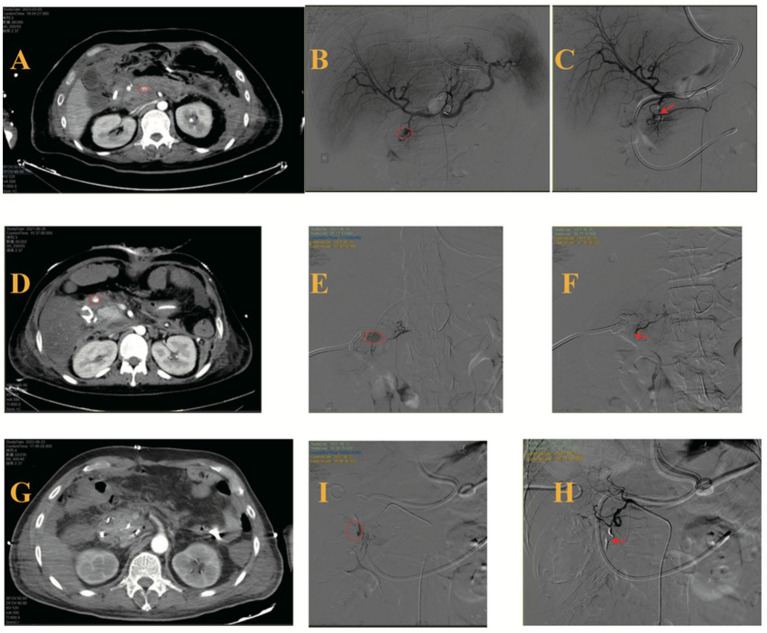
Representative imaging of severe acute pancreatitis (SAP)-associated hemorrhagic complications managed with digital subtraction angiography (DSA) and transcatheter arterial embolization. Red dashed circles indicate the bleeding sites, and red arrows indicate the embolization positions. Case 1: A 34-year-old man with idiopathic SAP developed intra-abdominal hemorrhage on day 63 after disease onset. (A) Contrast-enhanced CT (CECT) demonstrates a newly developed pseudoaneurysm arising from the gastroduodenal artery (GDA). (B) DSA confirms the pseudoaneurysm with active bleeding. (C) Post-embolization angiography demonstrates complete exclusion of the pseudoaneurysm without residual contrast extravasation. Case 2: A 22-year-old man with hypertriglyceridemia-associated SAP developed intra-abdominal hemorrhage approximately 2 months after distal pancreatectomy, splenectomy, and external drainage. (D) CECT demonstrates active hemorrhage adjacent to the duodenum. (E) Selective angiography of the superior mesenteric artery reveals persistent active contrast extravasation from a branch of the pancreaticoduodenal artery. (F) Post-embolization angiography confirms complete cessation of contrast extravasation following embolization with 100–300 μm gelatin sponge particles. Case 3: A 77-year-old man with biliary pancreatitis developed intra-abdominal hemorrhage during the postoperative period following surgical drainage for infected pancreatic necrosis complicated by severe retroperitoneal inflammation, gastrointestinal fistula formation, and temporary loop ileostomy. (G) CECT demonstrated no definite active contrast extravasation or newly formed hematoma. (H) Despite negative CECT findings, persistent clinical suspicion prompted DSA, which revealed active bleeding from a distal branch of the gastroduodenal artery (GDA). (I) Post-embolization angiography demonstrated successful hemostasis with complete occlusion of the culprit vessel after coil embolization.
